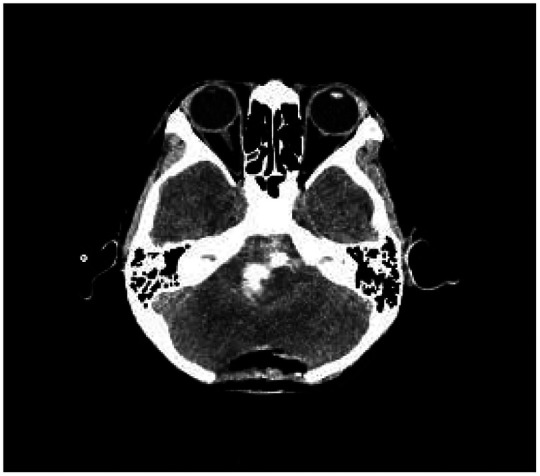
Postoperative CT shows hyperdense embolization material within the posterior fossa following endovascular treatment.
